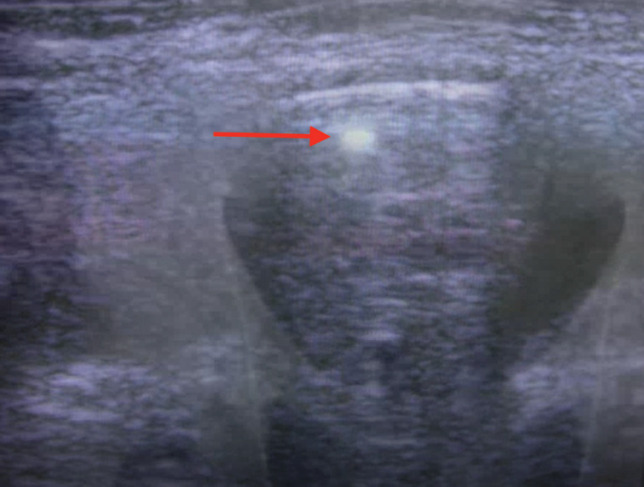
Arterial duplex ultrasound of the left leg. Red arrow shows the projectile in the common femoral artery
